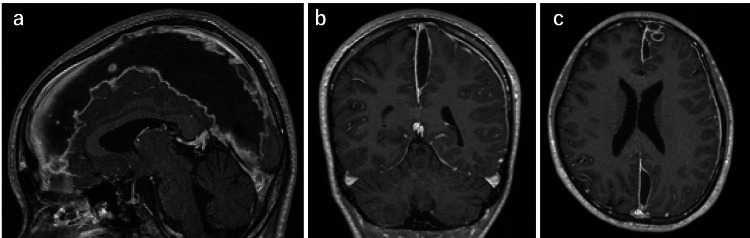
High-resolution 3D T1-weighted turbo field echo imaging of the brain. a) Sagittal, b) coronal, c) axial High-resolution 3D T1-weighted turbo field echo imaging of the brain shows a uniform low-signal area with a contrast effect under the dura along the left interhemispheric fissure. Extensive abscess formation was suspected.
